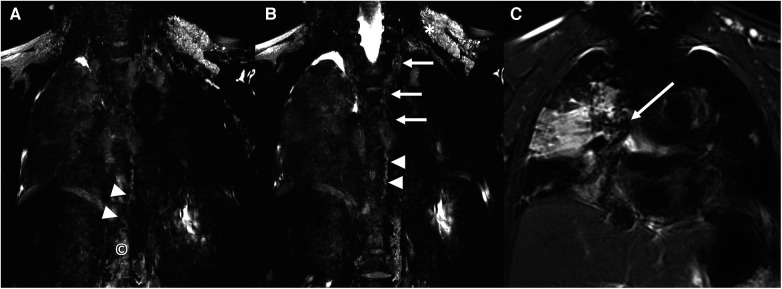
Coronal images from a three-dimensional, heavily T2-weighted MRI sequence (siemens magnetom vida 3 T). Cisterna chyli (A; ©) and the lower third of the thoracic duct (A,B; arrowheads) are demonstrated. The tubular aspect of the thoracic duct is lost in its middle and upper portions with an increased abnormal signal intensity at these levels (B; arrows). Increased abnormal signal intensity is also seen in the bilateral supraclavicular regions, in particular on the left side (B; *) extending into the mediastinum and with an interstitial pattern into the right lung (A–C), surrounding the right bronchus (C; arrow).
